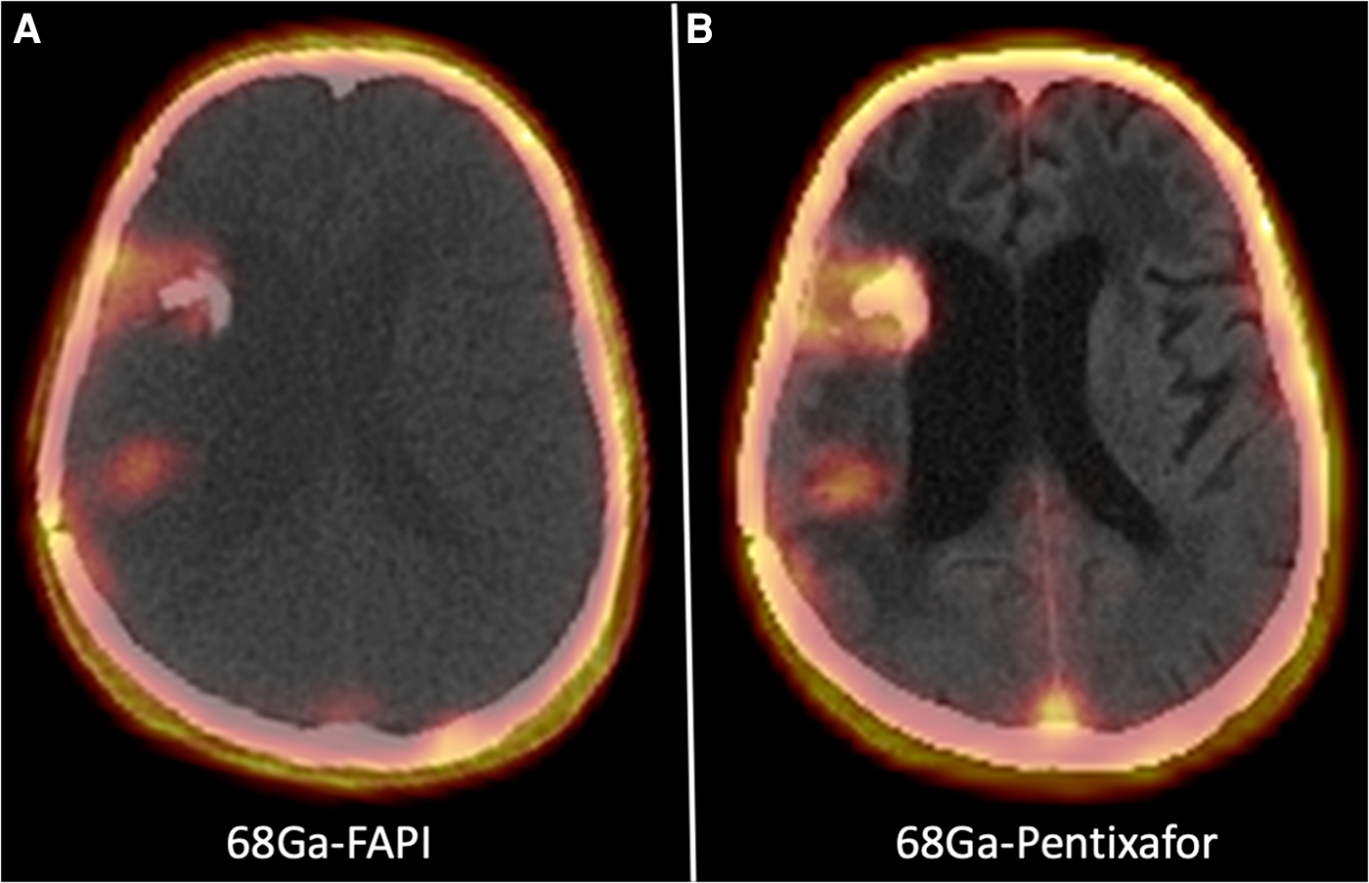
A 55-year-old patient with a history of GBM in the right frontotemporal lobe that underwent surgery and external beam radiation therapy and received ^177^Lu-DOTATATE. On ^68^Ga-Pentixather and ^68^Ga-FAPI imaging, there were three cerebral mass lesions in the right frontal and temporoparietal lobs, including a 11 × 15 mm lesion in the temporal lobe with SUV max = 4.15 (^68^Ga-Pentixather) and SUVmax = 4.154 (^68^Ga-FAPI), a 27 × 15 mm lesion in the temporal lobe (peri ventricular) with SUVmax = 1.97 (^68^Ga-Pentixather) and SUVmax = 2.68 (^68^Ga-FAPI), and a third lesion with a diameter of 12 × 14 mm in the right temporal lobe with SUVmax = 2.23 (^68^Ga-Pentixather) and 1.82 (^68^Ga-FAPI).
